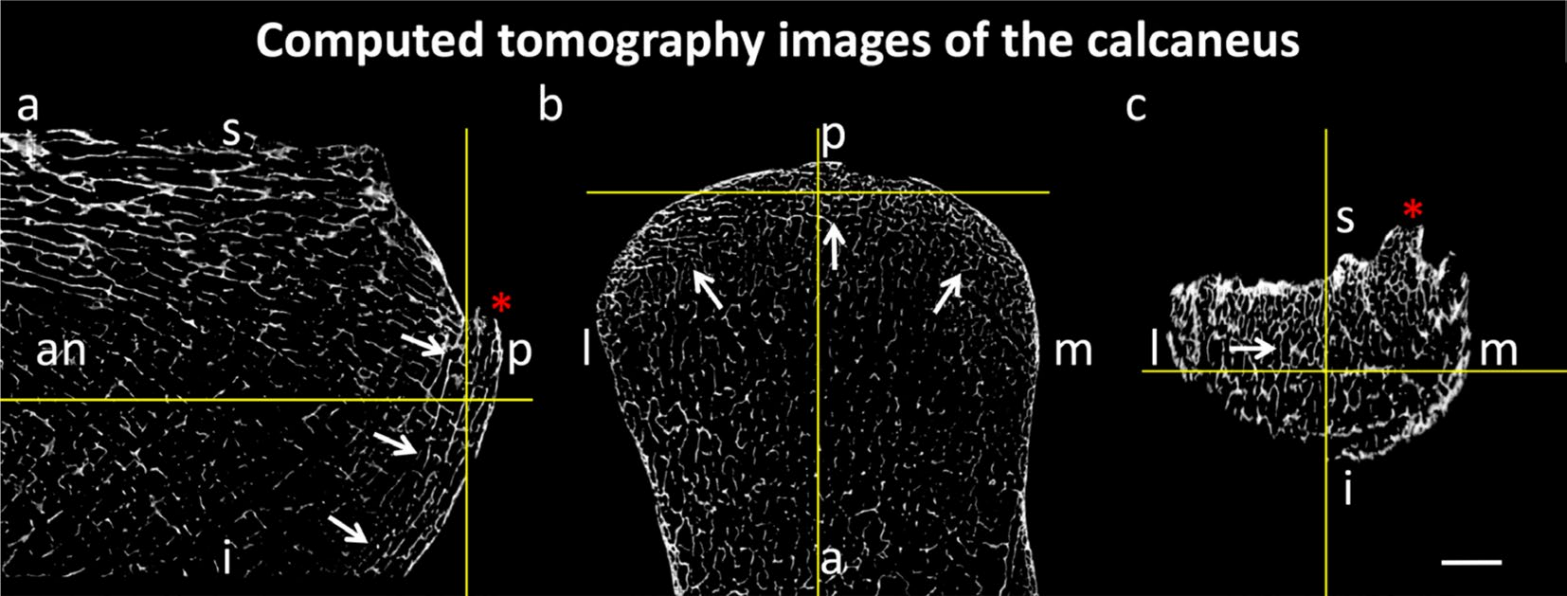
Computed tomography images of the calcaneus are depicted. The aligned dense trabeculae (white arrows) within the superficial posterior calcaneus are shown in a sagittal (a), transverse (b) and coronal (c) plane (white arrows). The center of the yellow cross marks the same spatial point within the three different planes. *an* anterior; i, inferior; *l* lateral; *m* medial; *p* posterior; *s* superior; red asterisk, calcaneal spur; scale bar: 5 mm.
